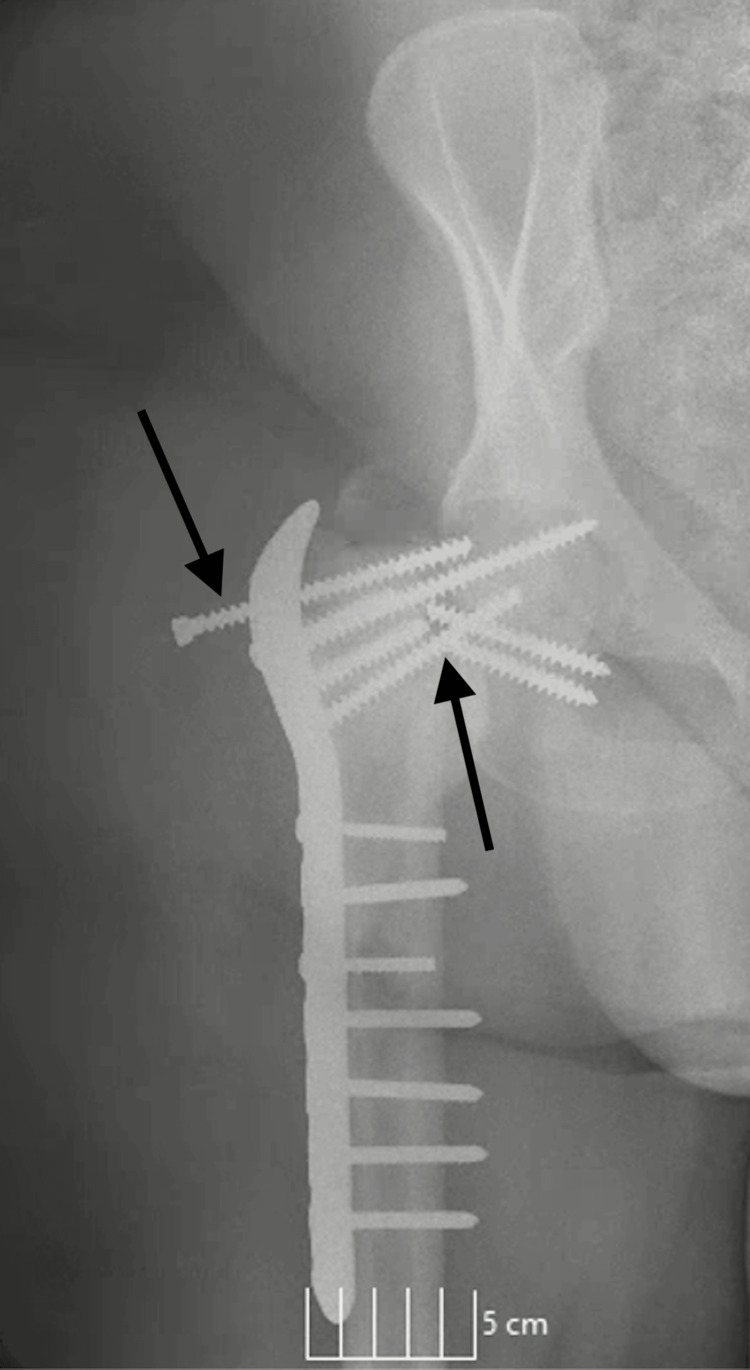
Post-ORIF revision X-ray following the fall - two broken screws traversing the femoral neck with significant retraction of one screw ORIF: open reduction and internal fixation
